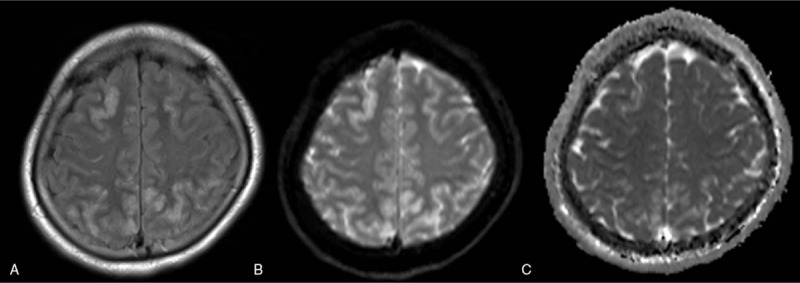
Radiographical findings on admission day. MRI axial T2-weighted FLAIR (A), DWI (B), and ADC mapping (C) demonstrated high-density areas in the parieto-occipital and frontal lobes. ADC = apparent diffusion coefficient, DWI = diffusion-weighted magnetic resonance imaging, FLAIR = fluid-attenuated inversion recovery, MRI = magnetic resonance imaging.
